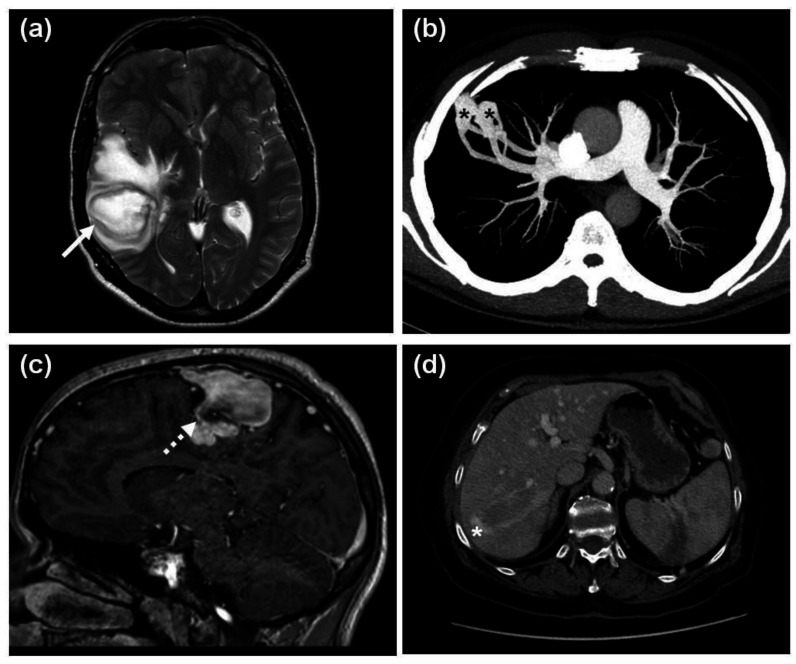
Visceral manifestations of HHT. (a) An axial plane, T2-weighted MR image of a cerebral abscess (white arrow) in the right temporal lobe of a 43-year-old HHT1 male and (b) the axial plane maximum intensity projection (MIP) CT image of the underlying 20 mm and 16 mm PAVMs (black asterisks) and their feeding vessels in the periphery of the right S3 segment in the same patient; (c) A 3DT1 postcontrast sagittal plane image of a CAVM (white dotted arrow) in the right parietal lobe of a 21-year-old male with HHT1; (d) An axial plane venous phase CT image showing a curved portovenous HAVM (white asterisk) with feeding vessels in the right liver lobe of a 73-year-old female HHT2 patient.
